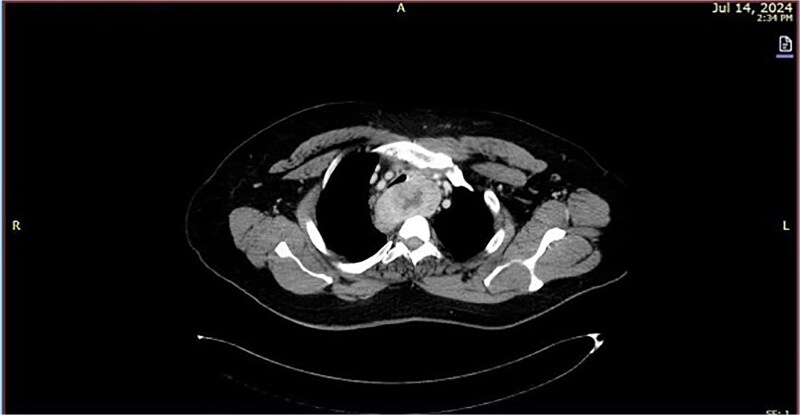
Transverse CT image showing a heterogeneous posterior mediastinal mass with central necrosis.
